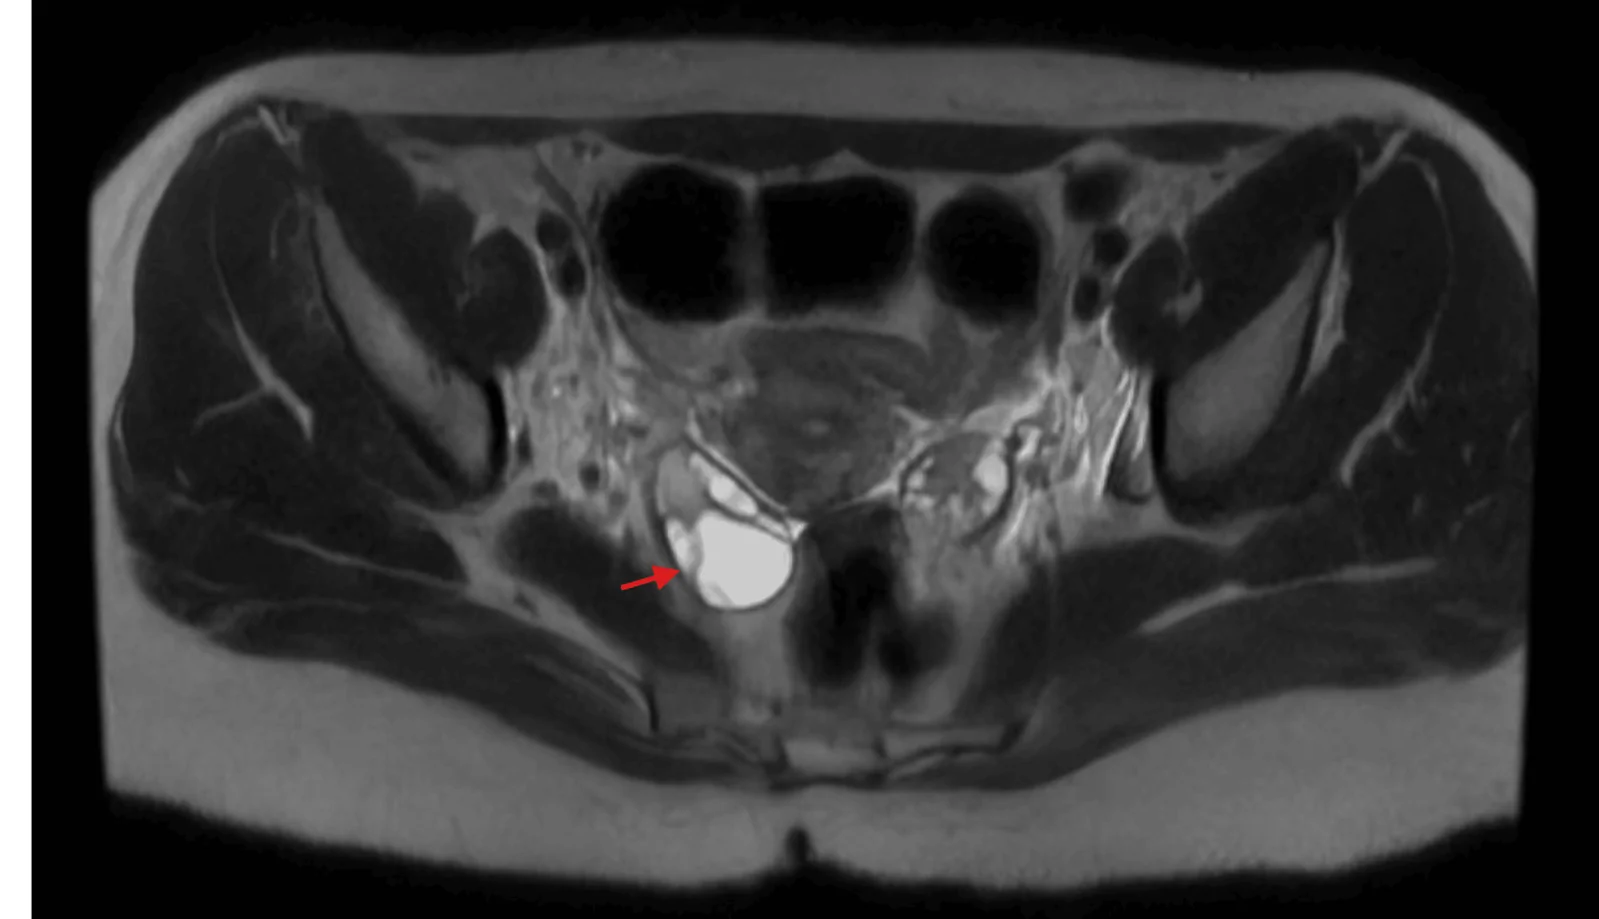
Contrast-enhanced MRI of the abdomen showing a 19×20 mm septated cyst in the right ovary, classified as O-RADS 3 (red arrow) MRI, magnetic resonance imaging; O-RADS, Ovarian-Adnexal Reporting and Data System
